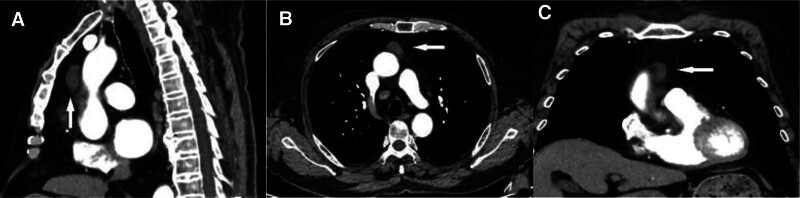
(A–C) Chest computed tomography shows a well-defined lesion in the anterior mediastinum (arrow), which exhibits no significant enhancement of its cystic component.
